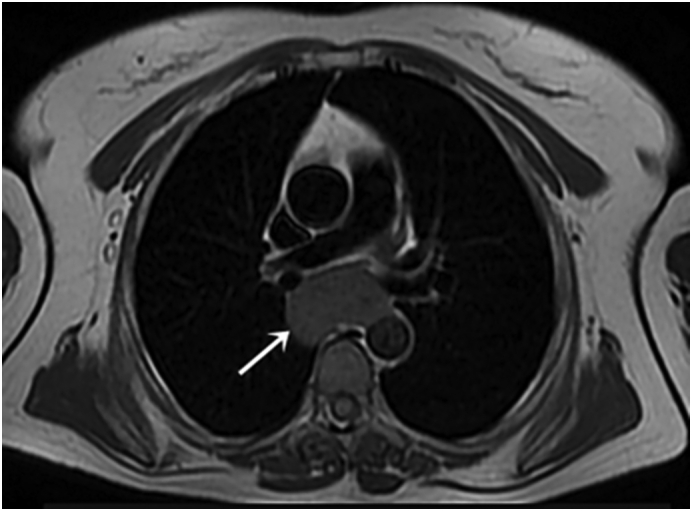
Chest MRI showing esophageal tissular mass (white arrow).
